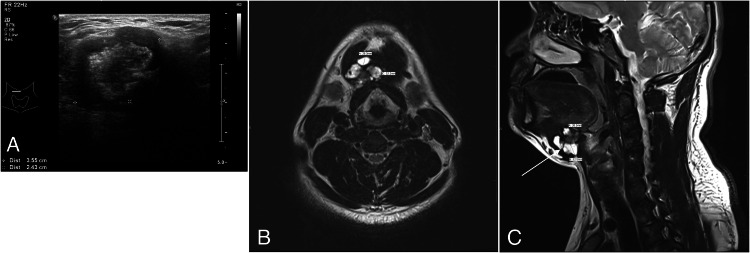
(A) US transverse image through the right paramedian thyrohyoid region shows a well-defined heterogeneous lesion with a peripheral thick hypoechoic wall and internal echogenic content. (B) Axial T2-weighted MRI of the neck shows a well-defined lesion with mixed signal intensity in the right paramedian region of the upper neck, anterior to thyrohyoid region with involvement of floor of mouth. It shows internal cystic areas, thick septations as well as solid hypodense components. (C) Sagittal T2-weighted MRI of the neck shows a well-defined mixed signal intensity lesion (arrow) in the anterior thyrohyoid region of the upper neck with involvement of floor of mouth. It shows internal cystic areas, thick septations, and solid hypodense components. US: Ultrasound, MRI: Magnetic Resonance Imaging
